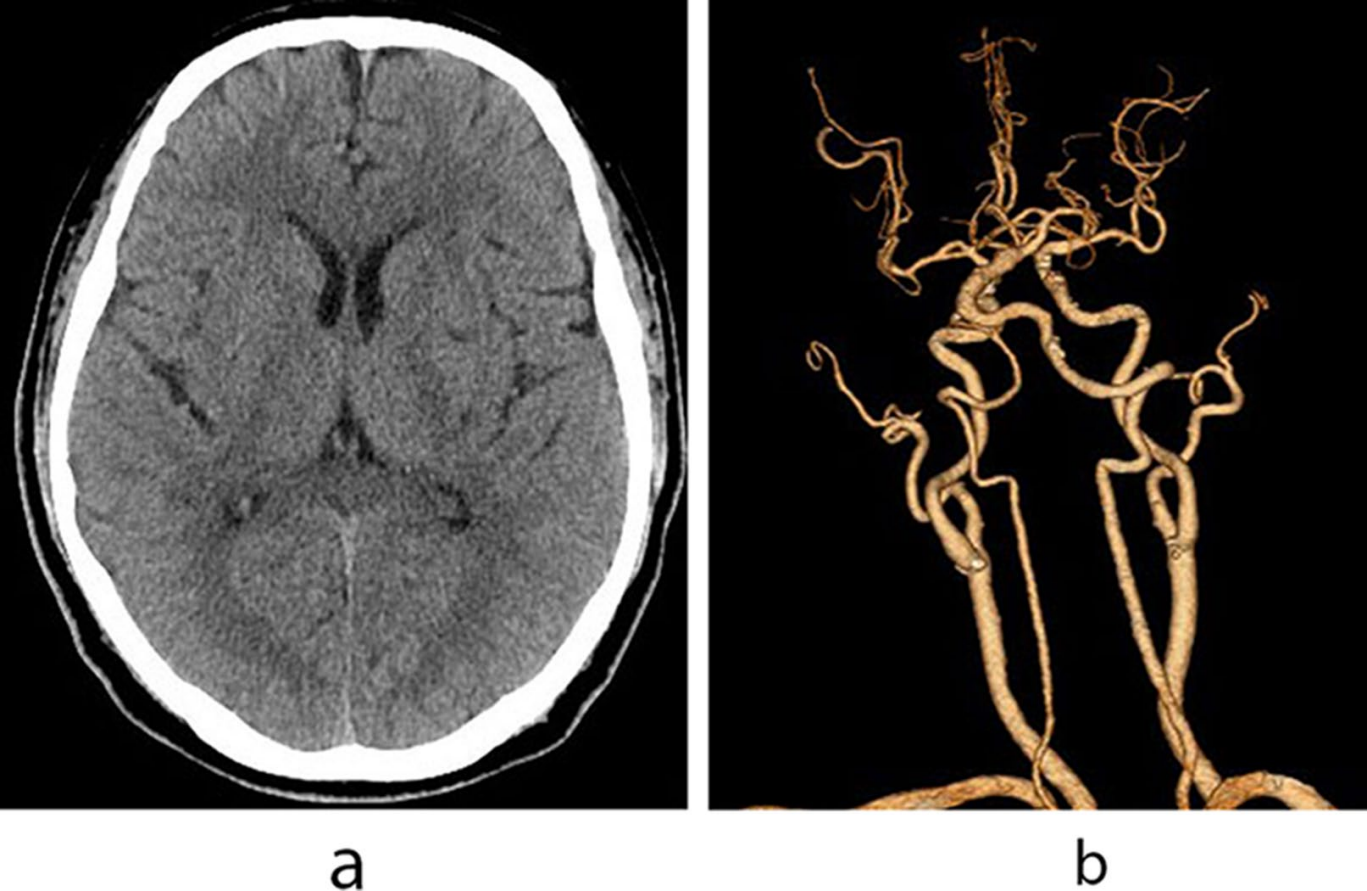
(A) Initial non-enhanced head computed tomography (CT) 3.3 h after stroke onset shows no intracranial hemorrhage. (B) CT angiography of the head and neck immediately after intravenous thrombolytic treatment shows no significant stenosis or occlusion of the carotid and major intracranial arteries except bilateral mild to moderate atherosclerosis of carotid and fenestration of the left proximal middle cerebral artery
